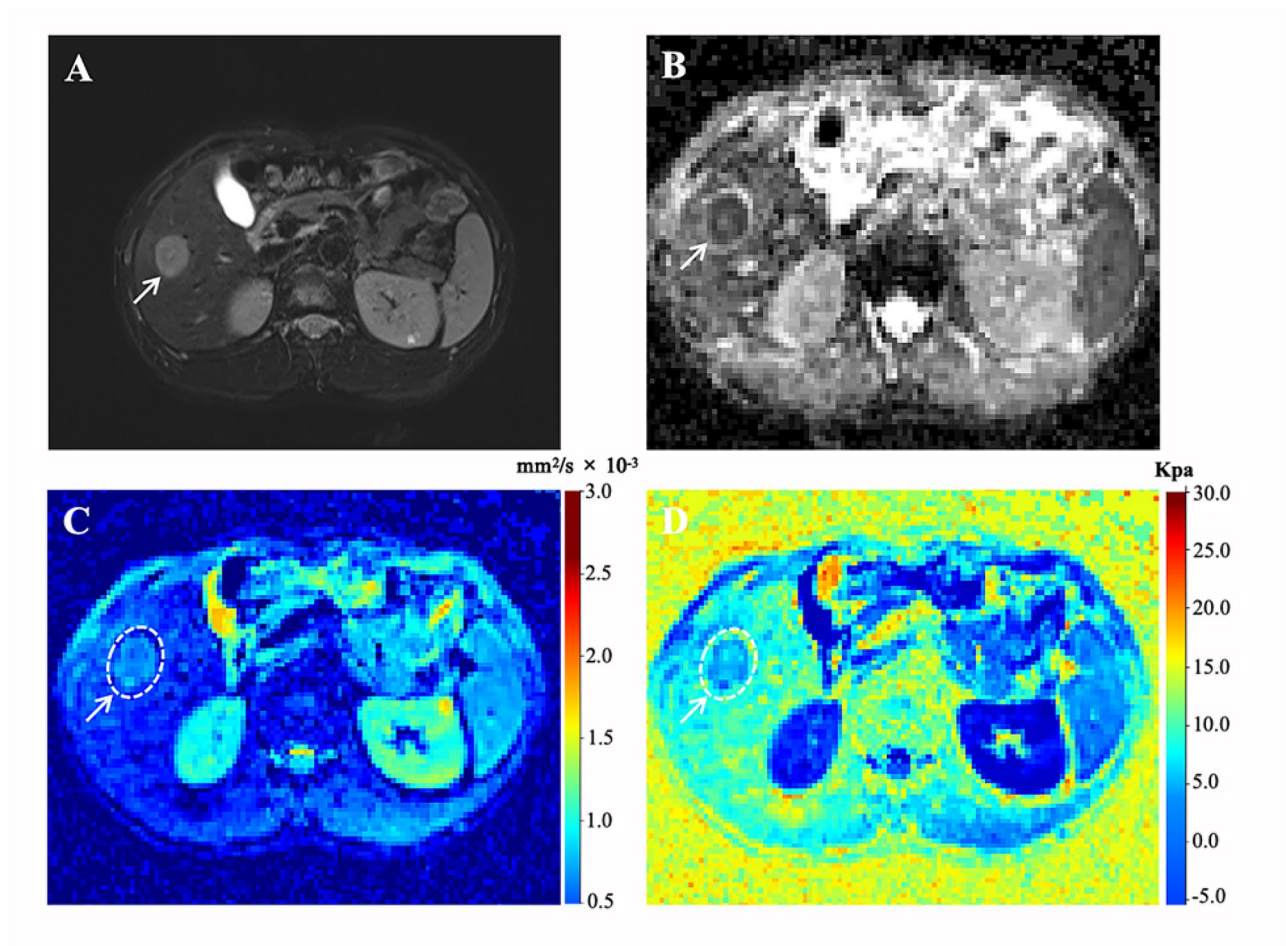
Representative MR images of a 47-year-old man with pathologically verified HCC. Tumor recurrence occurred 13 months after surgery. (A) Axial T2-weighted image showing a hyperintensity mass (arrow) on segment VI of the liver. (B-D) ADC, sADC, and *μ*_*diff*_ maps showing that the mean ADC, sADC, and μ_diff_ values of the tumor (arrow) were 1.44 × 10^− 3^ mm^2^/s, 0.70 × 10^− 3^ mm^2^/s, and 5.10 kPa, respectively. ADC, apparent diffusion coefficient; sADC, shifted apparent diffusion coefficient; *μ*_*diff*_, DWI-based virtual shear modulus
